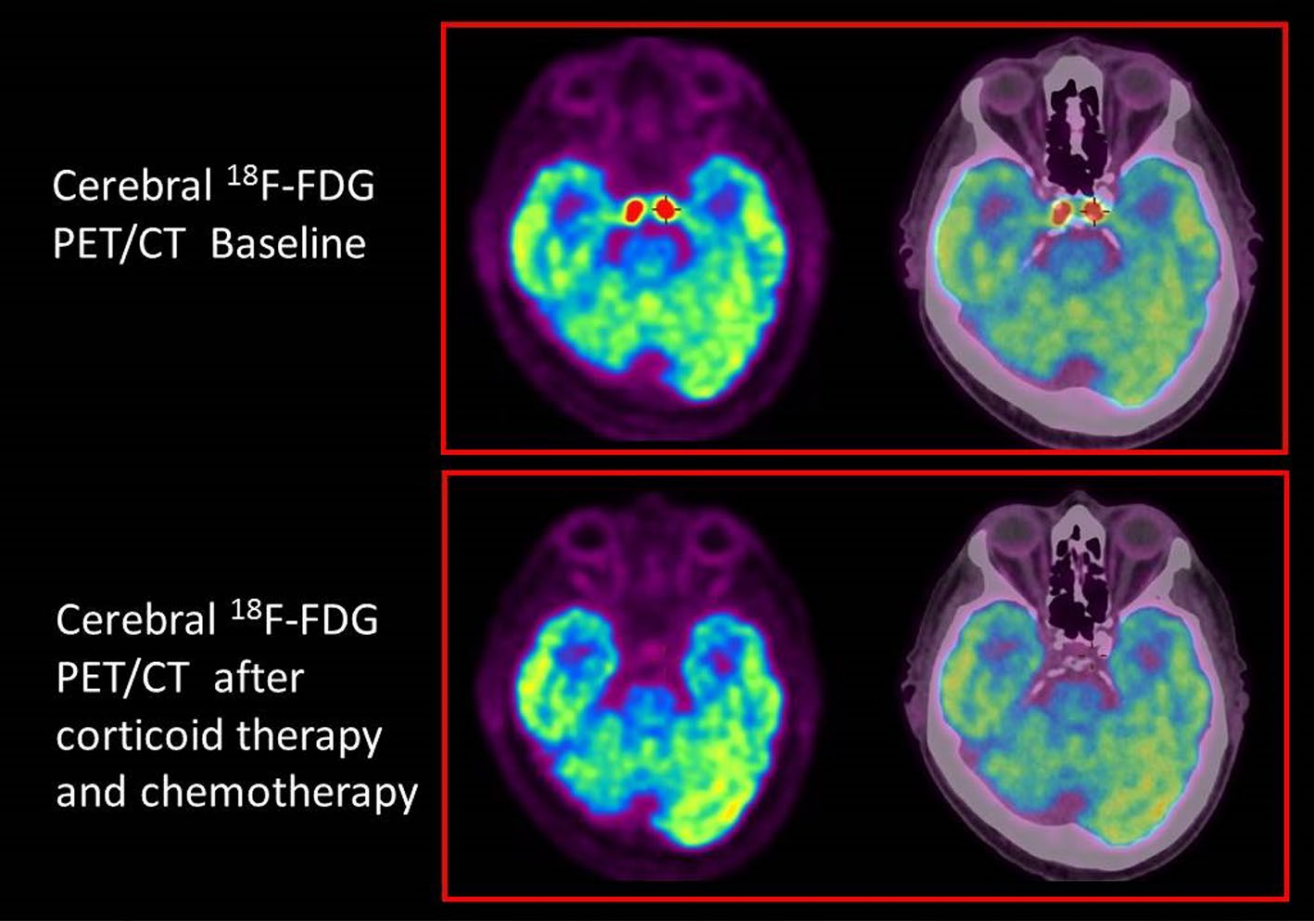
Cerebral ^18^F-FDG PET/CT demonstrating a complete response after corticoid therapy, 6 cycles of R-CHOP and 4 cycles of HD-MTX
